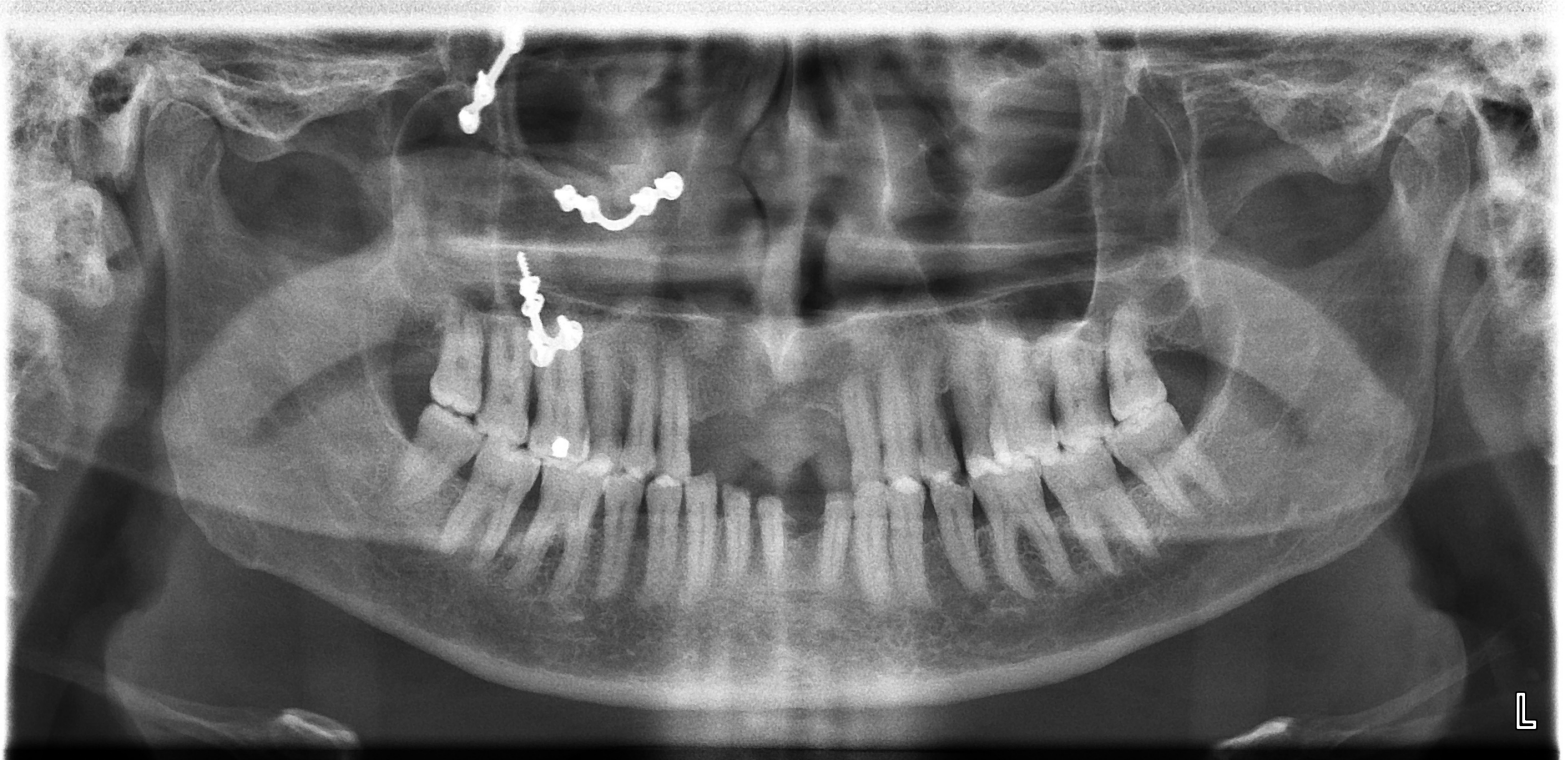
Radiographic investigation
